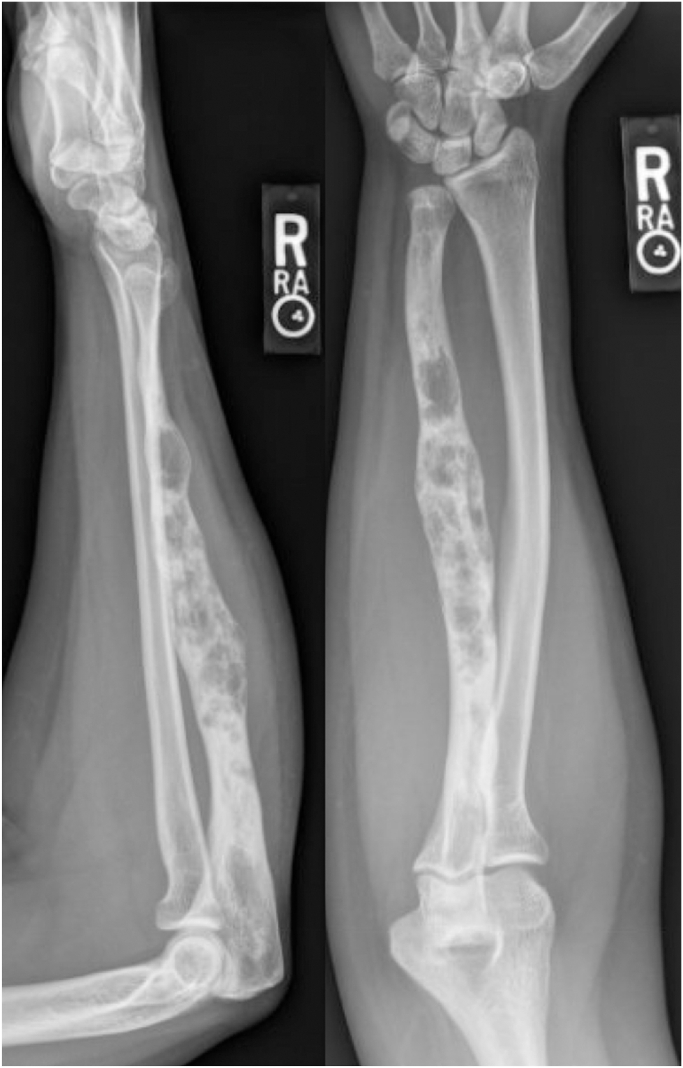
Patient at age 26. Radiographs demonstrated an enlarged ulnar shaft with a mixed lytic and blastic lesion involving greater than 90% of the cortical width.
